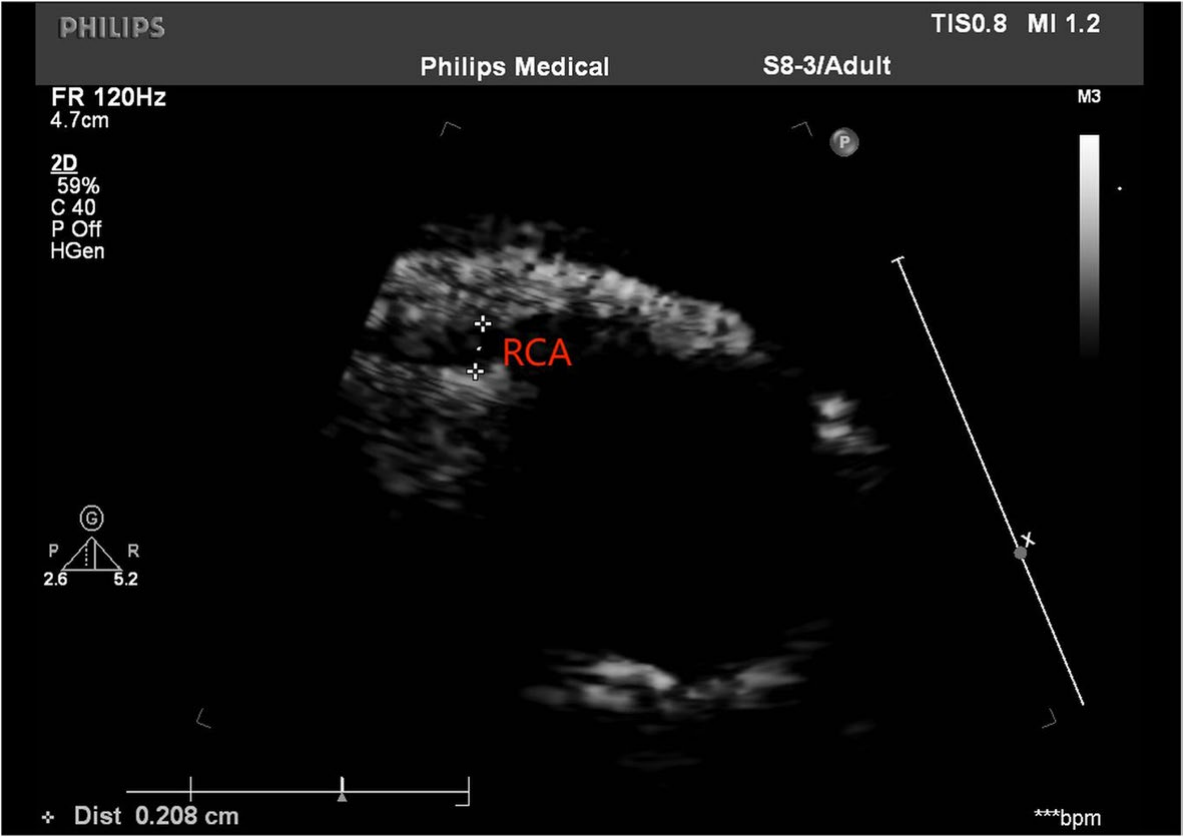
Ultrasound measurement of the right coronary artery
